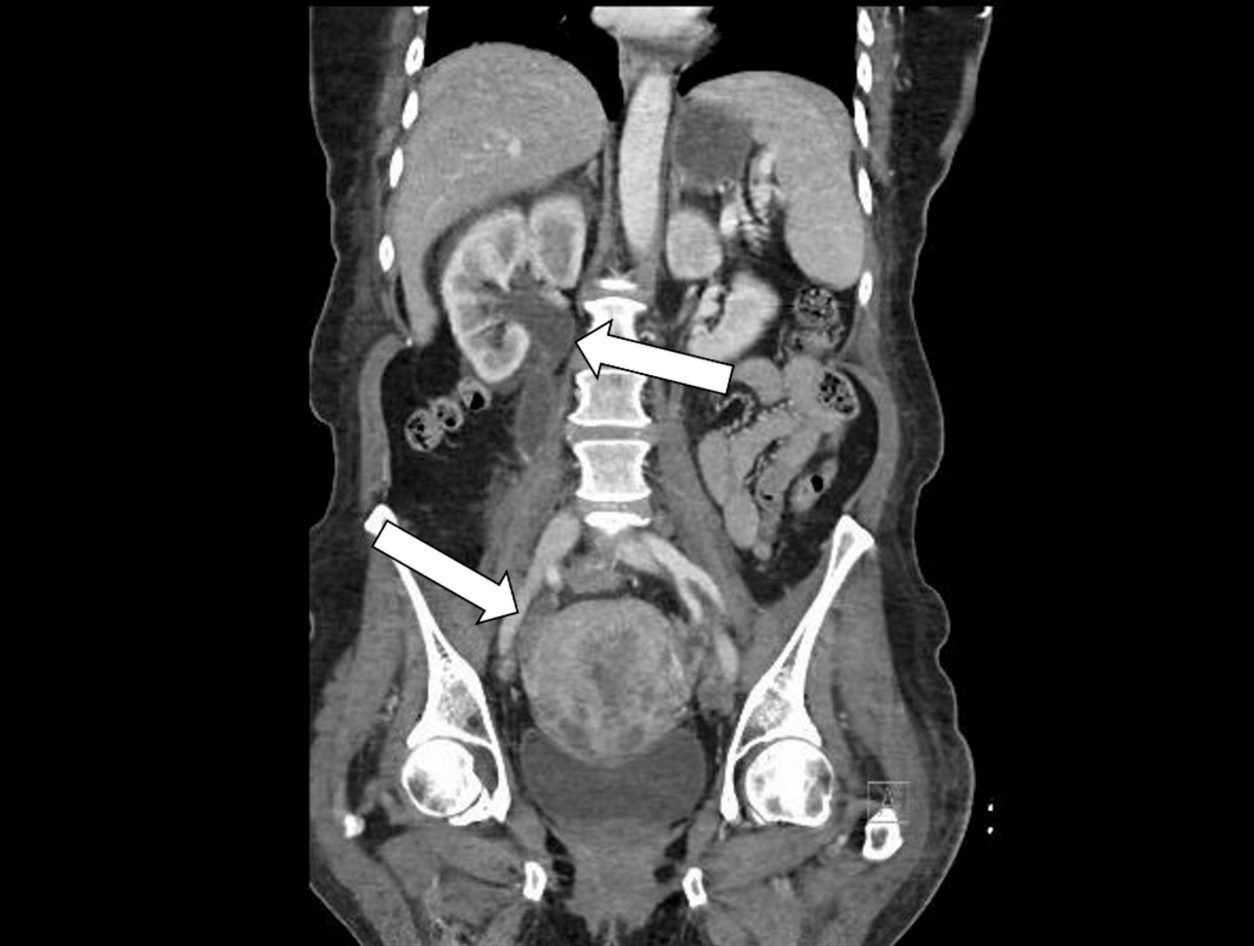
Computed tomography of the abdomen/pelvis with contrast. The image reveals right-sided hydronephrosis secondary to obstruction from nodular uterine mass (arrows).
